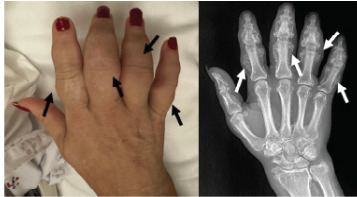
Left: Several non-mobile hard lesions on the right hand associated with interphalangeal joint swelling (black arrows). Right: Radiograph revealing periosteal new bone formation throughout the right hand (white arrows).
